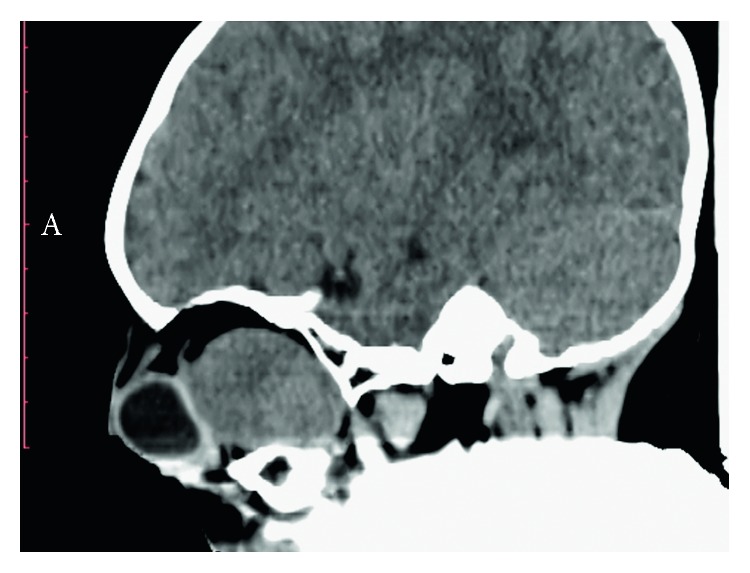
CT scan showing the orbital involvement with the lateral wall of the orbit infiltration.
